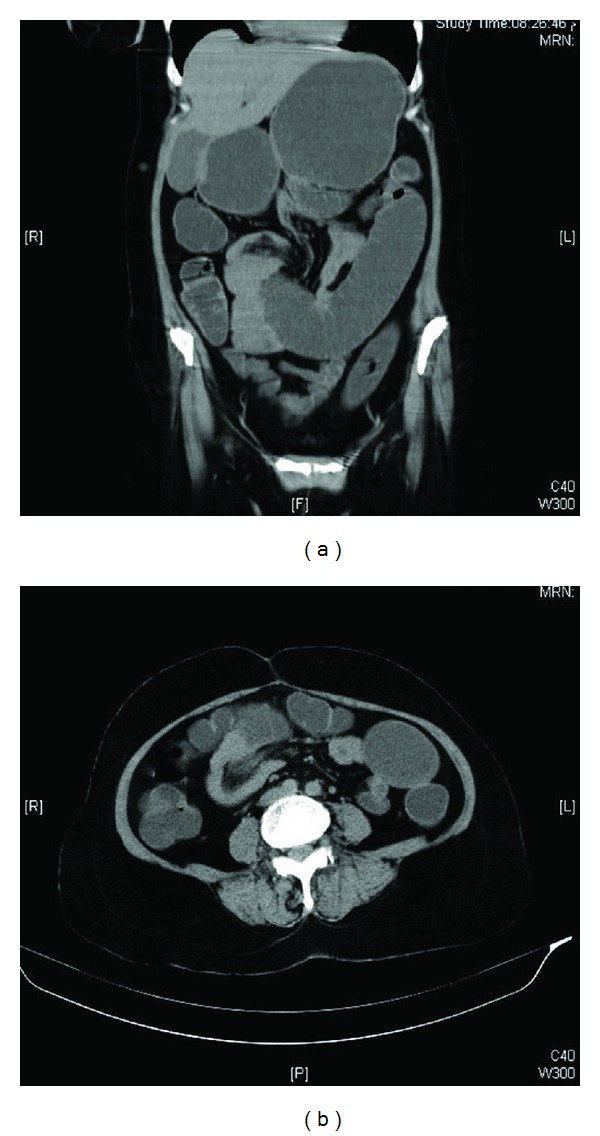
Computed tomography (CT) abdomen without contrast showing (a) coronal view: distended stomach, proximal small bowel loops extending down to midabdominal level where there was abrupt change in caliber of the bowel, and collapse of distal small bowel loops and (b) axial view: a soft tissue density lesion containing vessels.
